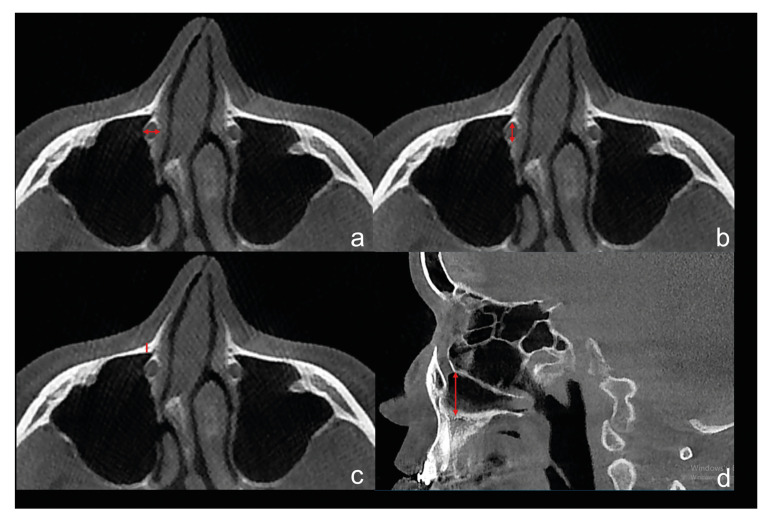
Measurement of the end of the bony nasolacrimal canal (inferior level) on preoperative CBCT images a: The mediolateral diameters of a bony nasolacrimal canal in the section where the canal ends in the axial plane, b: The anteroposterior diameter of the bony nasolacrimal canal, c: The distances between the anterior border of the nasolacrimal canal and the outer cortical surface of the maxilla, d: The distance between most inferior cortical border of nasolacrimal canal and the nasal floor.
